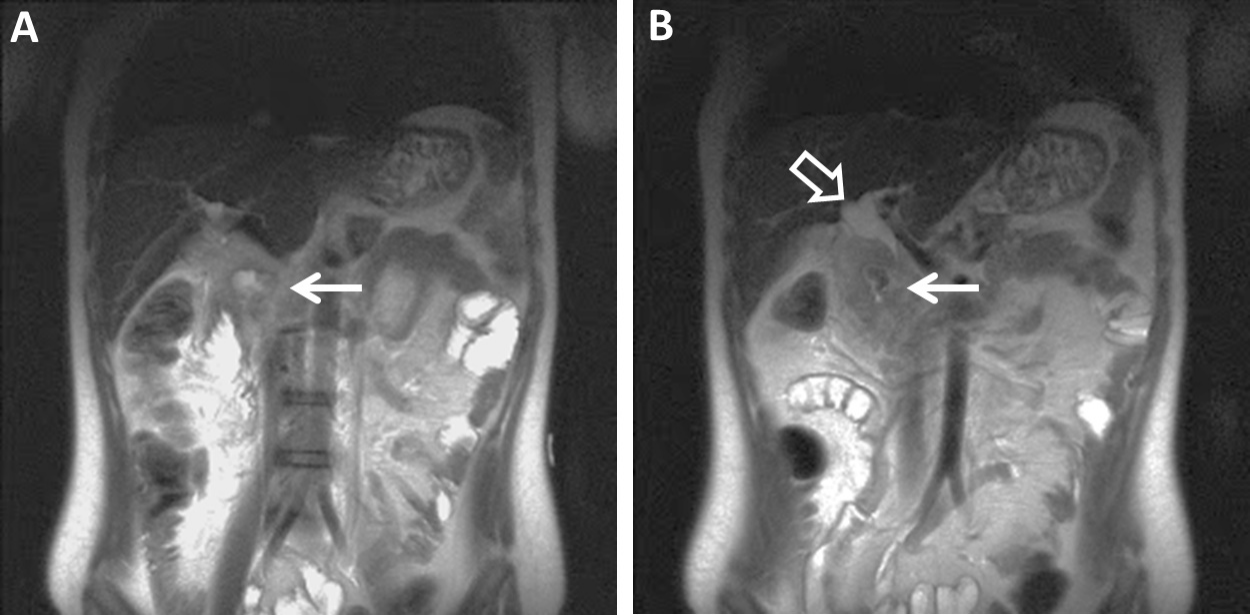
T2-weighted Coronal MRI of the Abdomen (Pre-Treatment) T2-weighted coronal MR images of the abdomen re-demonstrate (A) a periampullary duodenal diverticulum with surrounding inflammatory changes (arrow).  (B) A more posterior slice demonstrates a dilated common bile duct and proximal intrahepatic ducts (open arrow).
